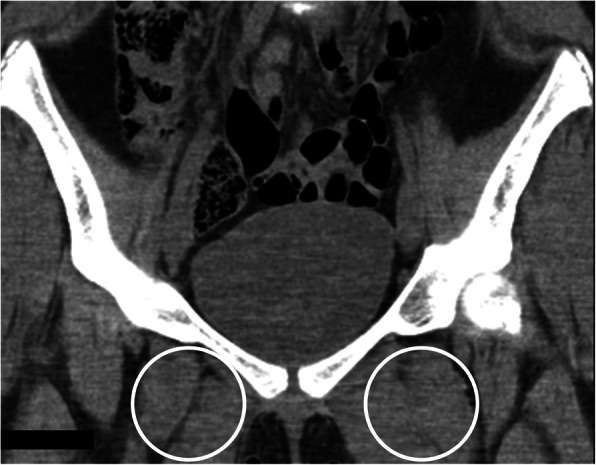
Coronal CT image from the same follow-up examination as Fig. 3 shows resolution of the areas of calcification in the adductor musculature (while circles
